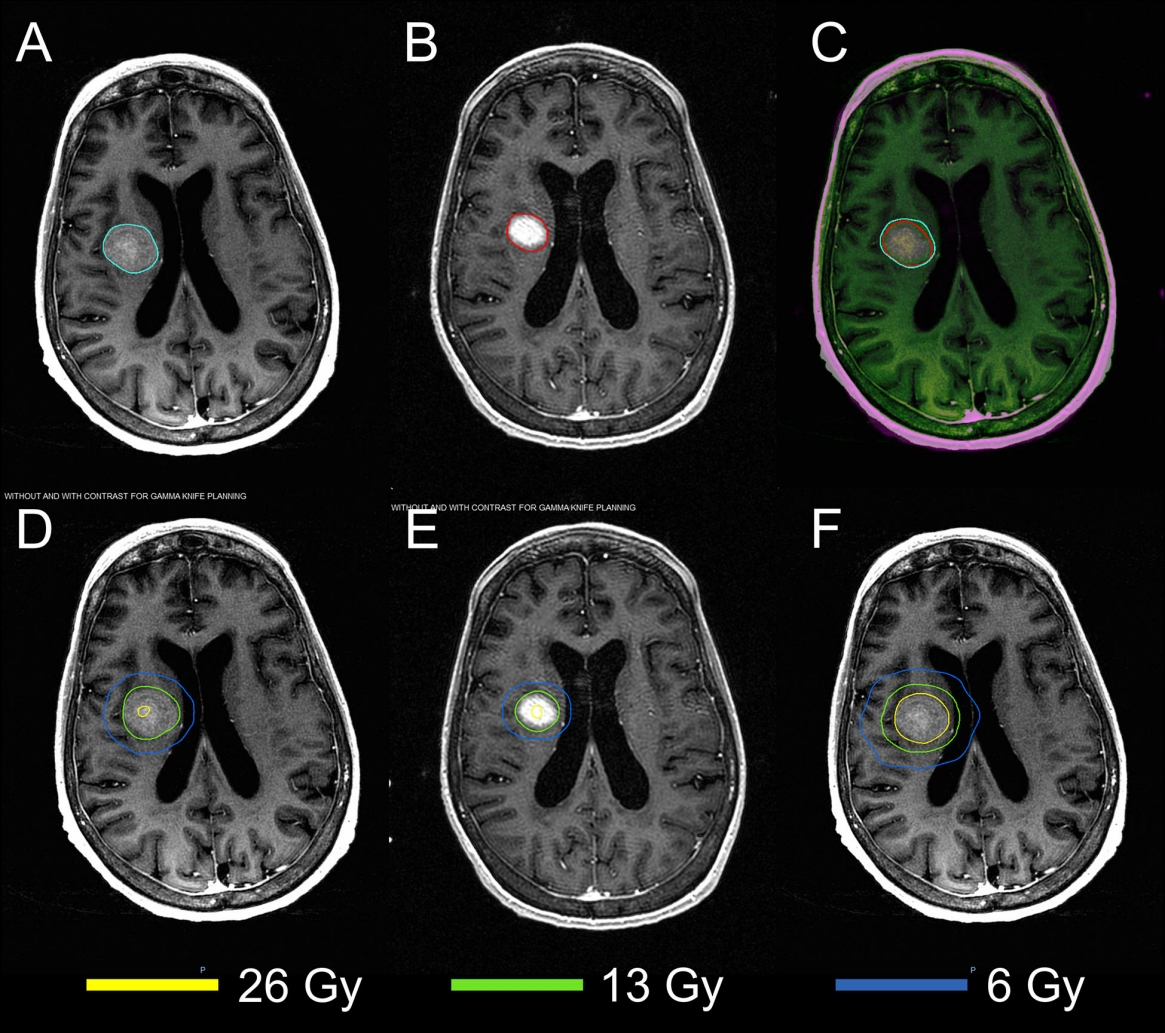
Demonstration of the dose summation for a representative case undergoing 2-stage GK SRS. Deformable image registration was performed to register the MRI acquired in the second session (B) to that acquired in the first session (A). The registration accuracy was evaluated by visually examining the MRI fusion (C) and by computing the Dice similarity coefficient between the original tumor contour (cyan) on the first MRI and the deformed tumor contour (red) on the second MRI. With the same deformation, the original dose distribution in the second session (E) was deformed and added to that in the first session (D). After the dose summation, thetotal dose distribution of the 2-stage GK SRS could be assessed on the first MRI (F).
